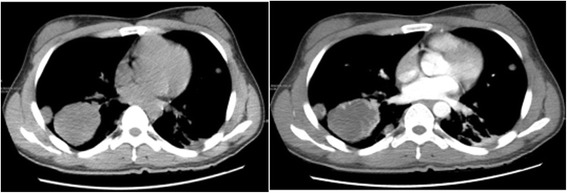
The primary CT showing multiple nodules and masses within the abdomen. The largest measures 5.7 × 6.0 cm
